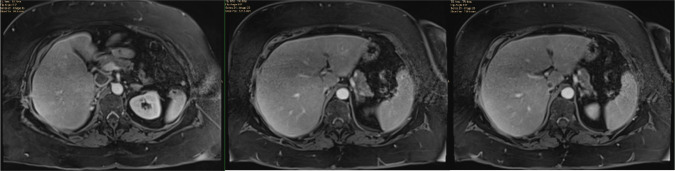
Pre-operative MRI showing tumor thrombus extending into the IVC below the major hepatic veins.
